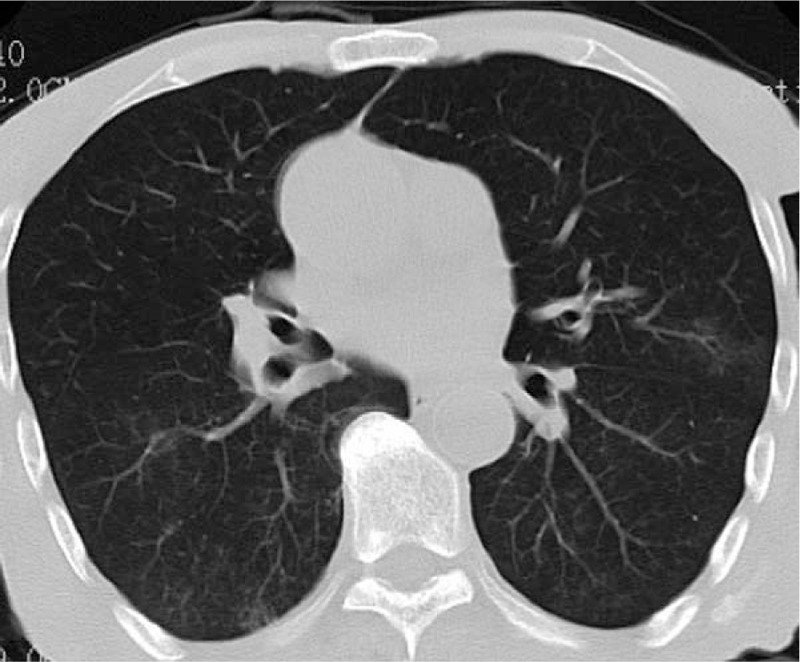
Chest computed tomography scan 6 months after olanzapine withdrawal, showing the resolution of the eosinophilic pleural effusion.
